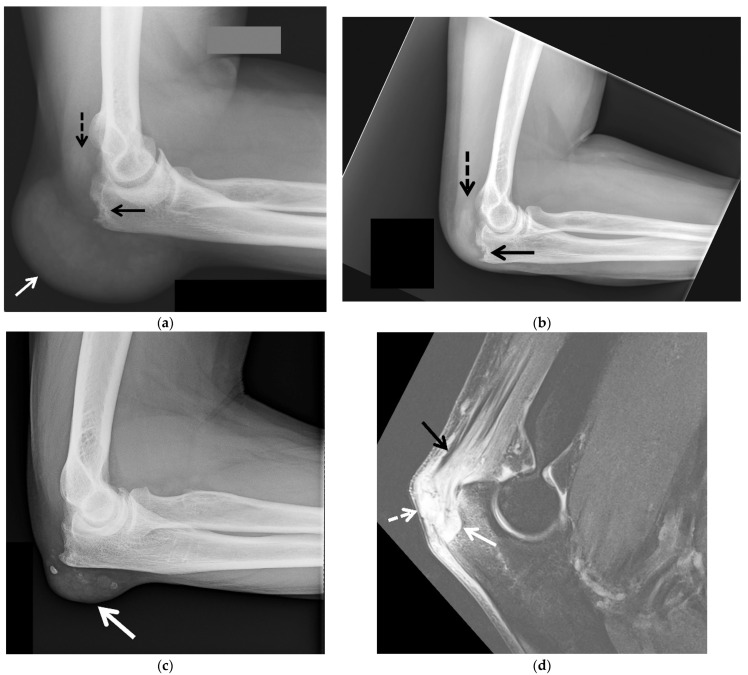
Tophaceous gout of the posterior elbow in 3 different patients. (a) Initial lateral radiograph of the left elbow in a 57-year-old man shows cortical erosion of the posterior olecranon (black arrow) with marked distension and somewhat increased density of the overlying olecranon bursa (white arrow). Note increased density of the distal triceps tendon (dashed black arrow). (b) Lateral radiograph of the left elbow of the same patient obtained after surgical debridement redemonstrated cortical erosion at the posterior olecranon (black arrow) and increased density of the distal triceps tendon (dashed black arrow) with interval marked improvement of posterior soft-tissue thickening. (c) Lateral radiograph of the right elbow in a 62-year-old man shows a soft-tissue mass involving the olecranon bursa with associated calcifications (arrow). (d) Sagittal T2-weighted with fat saturation MR image in a 60-year-old man shows a large bone erosion involving the posterior olecranon (white arrow) with associated mild bone marrow edema subjacent to a markedly thickened and irregular distal triceps tendon of heterogeneous increased signal intensity (black arrow). Note mildly distended irregular shaped, heterogeneous, predominantly high-signal-intensity olecranon bursa extending into the distal triceps tendon (white arrow) and additional high-signal-intensity subcutaneous edema at the posterior aspect of the elbow.
